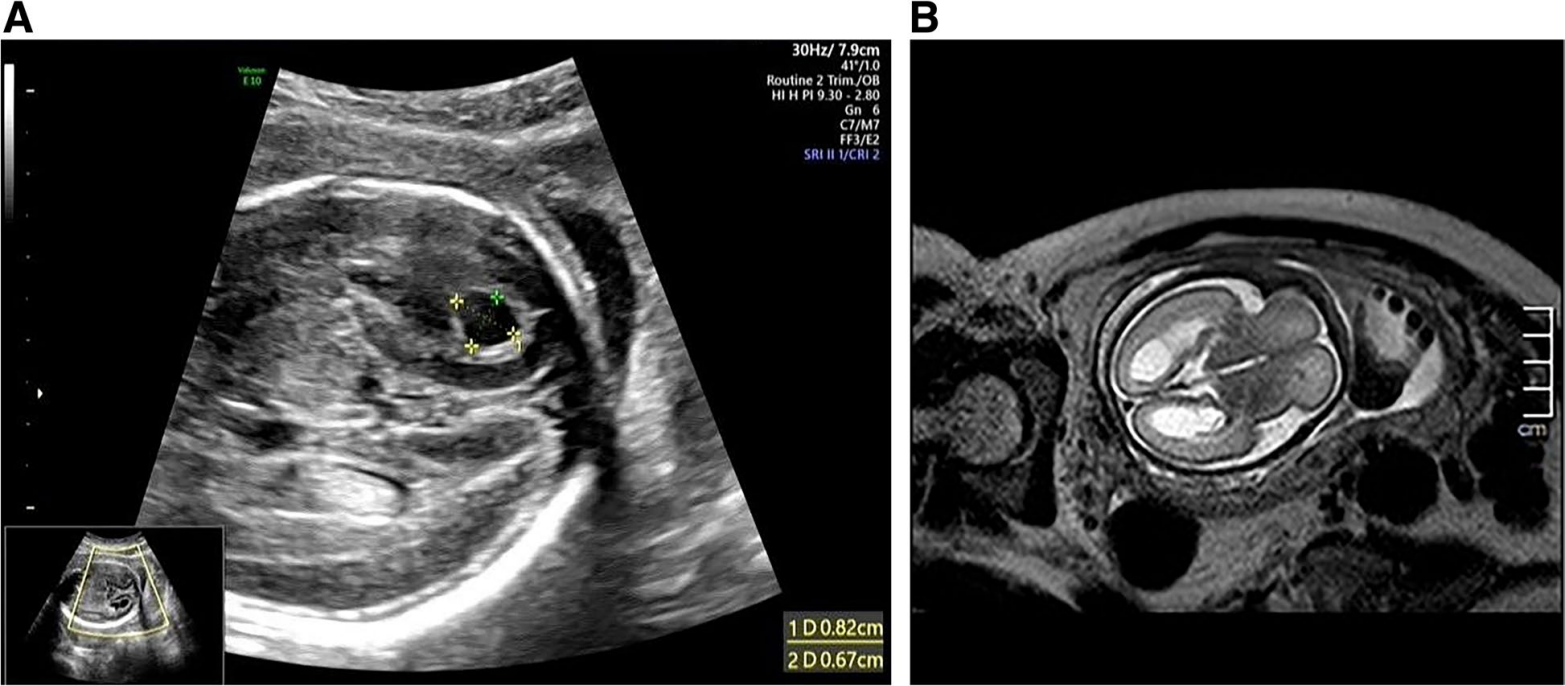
Prenatal ultrasound (A) and prenatal fetal MRI (B) showed cystic structures in the posterior horn of the fetal lateral ventricle
